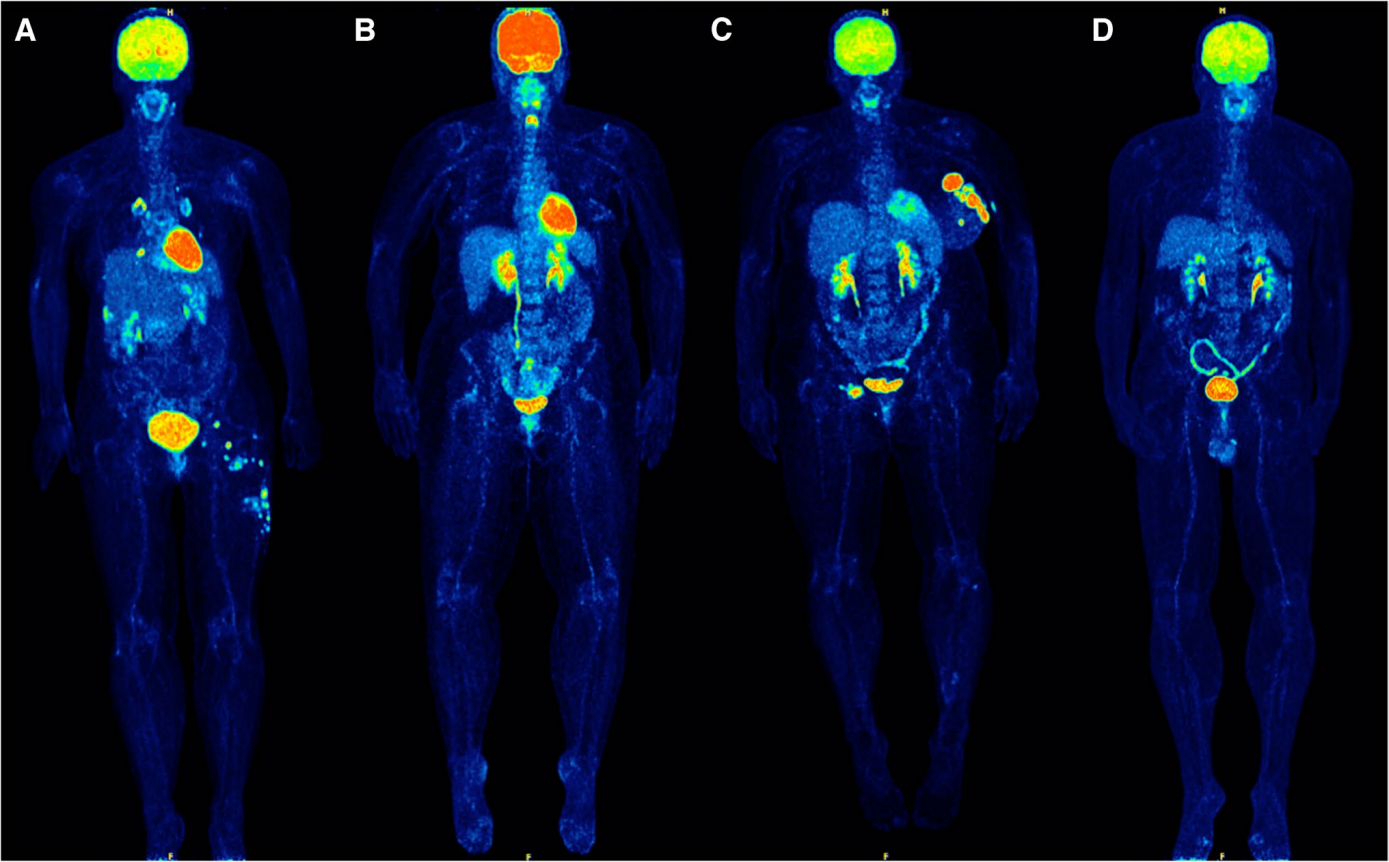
PET/CT examinations (maximal intensity projection images) of melanoma patients before initiation of ipilimumab-based immunotherapy, exhibiting different levels of colonic [.^18^F]FDG uptake. Based on visual assessment, colon uptake can be graded according to a four-point scale, as proposed by Gontier et al. [45]: 1. uptake less than the background hepatic activity (A), 2. uptake similar to that of the liver (B), 3. uptake moderately greater than that of the liver (C), 4. intense and diffuse uptake, markedly higher than hepatic activity (D)
